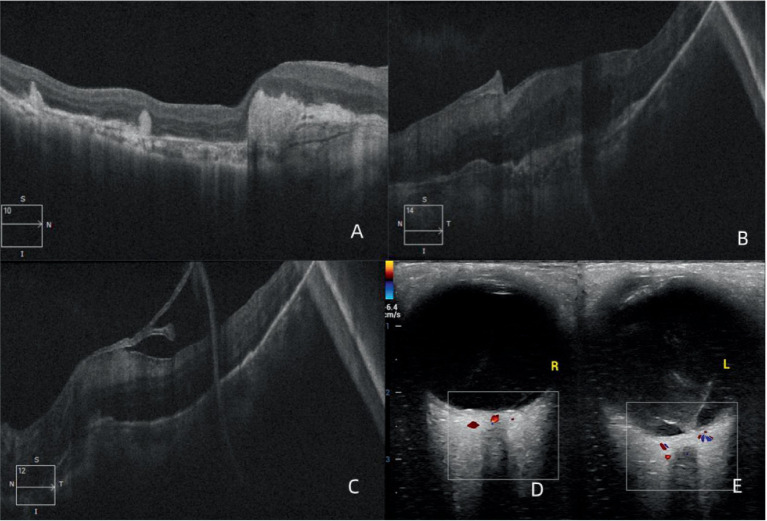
Binocular optical coherence tomography (OCT) and ocular B-ultrasound findings. (A,B) Macular OCT examination of the right and left eye, respectively; (C) OCT of the left eye shows the presence of vitreoretinal traction; and (D,E) ocular ultrasonography of the right and left eye, respectively.
